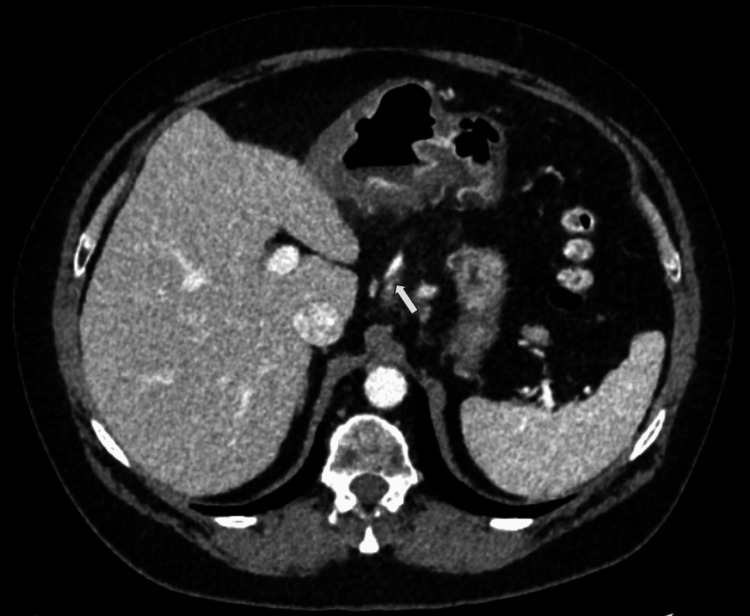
Axial CT of the abdomen and pelvis with contrast shows proximal subtotal occlusion (white arrow) and distal enhancement of the left gastric artery, which suggests high-grade, flow-limiting thrombotic occlusion. CT: computed tomography
